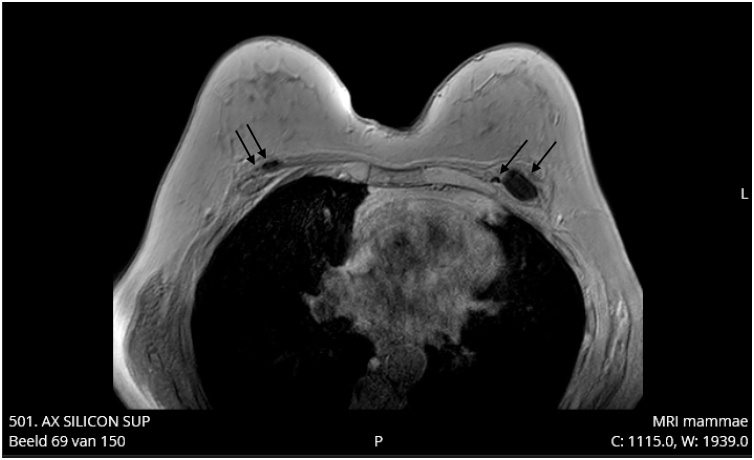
Silicone residuals after explantation of the breast implants on protocol breast MRI. The diameter of the largest silicone pocket (left) is 2.3 × 1.5 cm.
